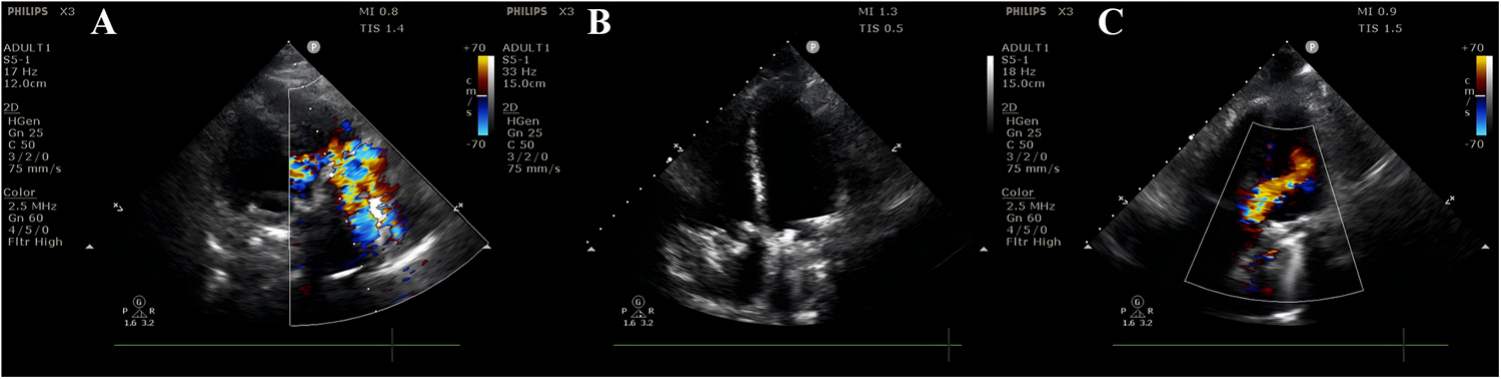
Postpartum cardiac ultrasound findings on day 3. (A) Mechanical tricuspid valve with significantly accelerated anterograde flow (Vmax = 2.5 m/s, PGmean = 14 mmHg) and severe regurgitation at the prosthetic orifice. (B) Mechanical tricuspid valve with stable frame; both leaflets exhibit semi-open positions (one leaflet shows no significant motion, while the other has restricted mobility). Hypoechoic echoes adherent to the valve surface (suspected thrombus or other etiology?). (C) Mechanical mitral valve with mildly accelerated anterograde flow. No definitive perivalvular regurgitation is observed. Color Doppler demonstrates clear flow signals without abnormal perivalvular echoes.
